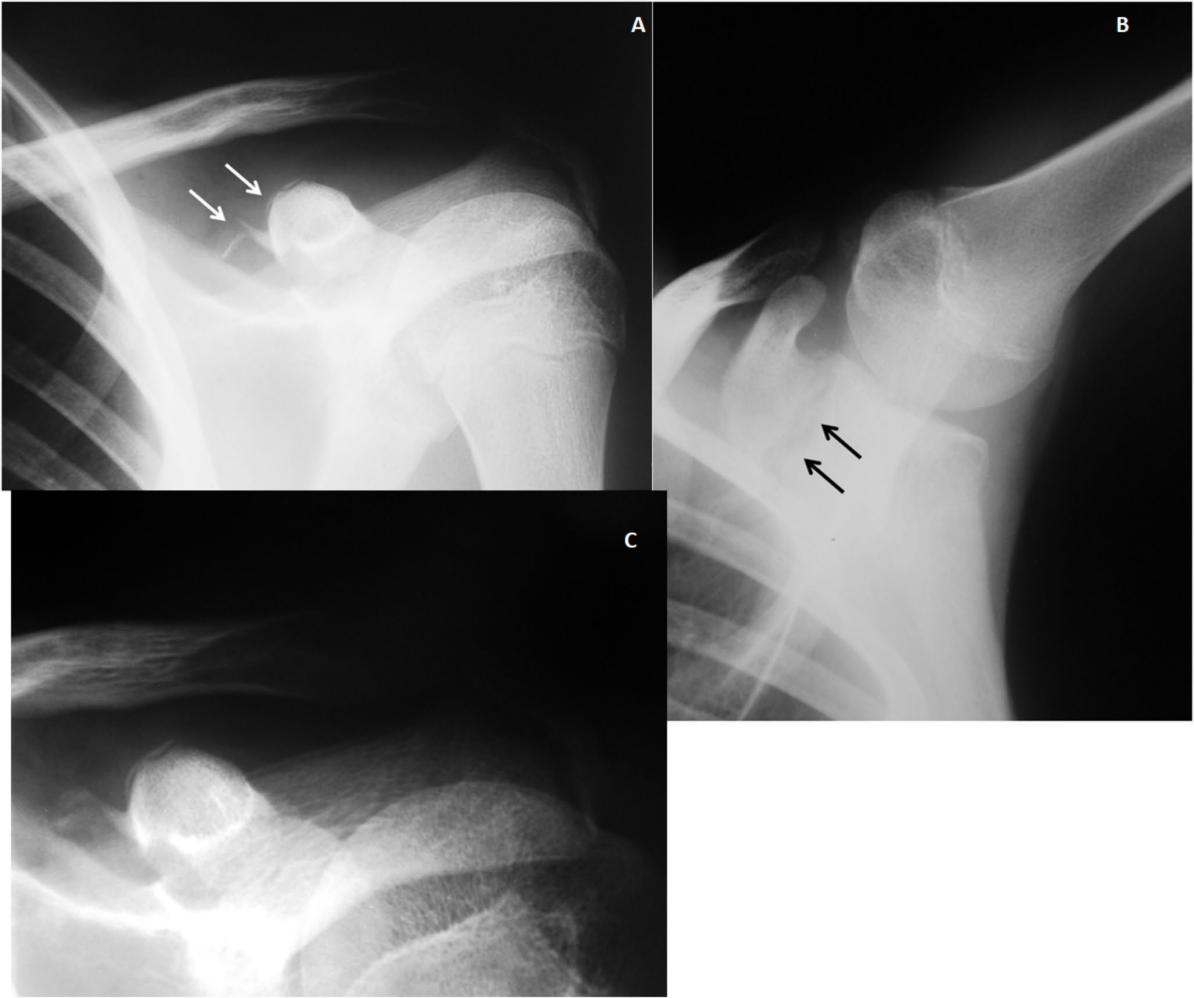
Case 8 Table 1. A 15 years-old boy: 3A: Antero-posterior radiograph showing an AC dislocation associated with a fracture of the superior border of the scapula that suggested a CP base fracture (white arrows); 3B: An abduction view radiograph reveals a fractured CP (black arrows); 3C: Conservative treatment by mean of Robert-Jones bandage was performed with good clinical result
